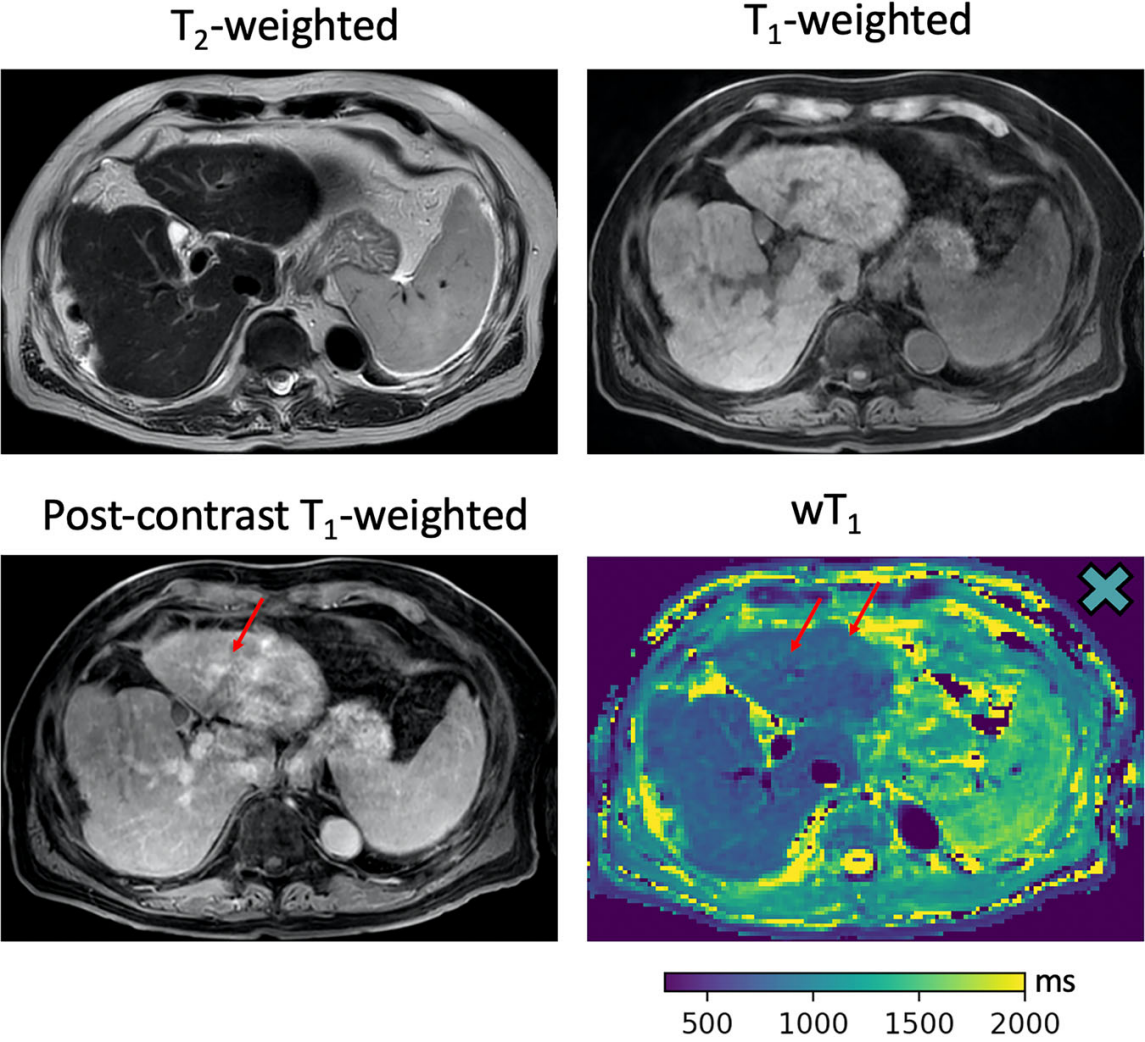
A 77-year-old male with multifocal carcinoma. No hepatic alterations are seen on unenhanced T2- and T1-weighted images. After contrast administration, qualitative fibrosis signs appear in S3 (arrow), while wT1 maps detect scars (arrows) even without contrast application. The wT1 and wT1_comp_ values of S3 (wT1_S3_ = 913 ms, wT1_comp-S3_ = 960 ms) are represented as a thick-edged X (right upper corner wT1 map) in Fig. 4c. wT1, Water T1; wT1_comp_, T2*-compensated wT1
